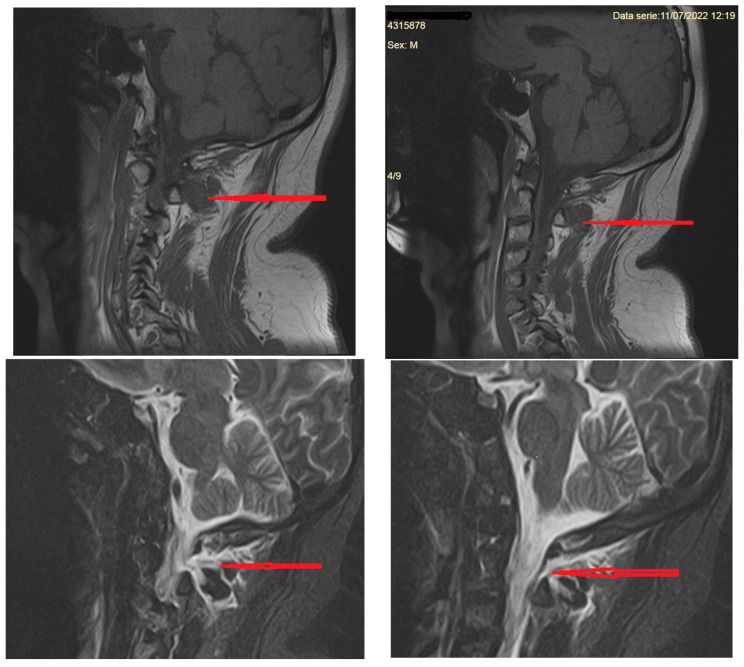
MRI of the cervical spine (T1 FSE sequences in the upper row and T2 STIR sequences in the lower row)) showing the fluid collection infiltrating paravertebral structures at the C1–C2 vertebral level (red arrows).
